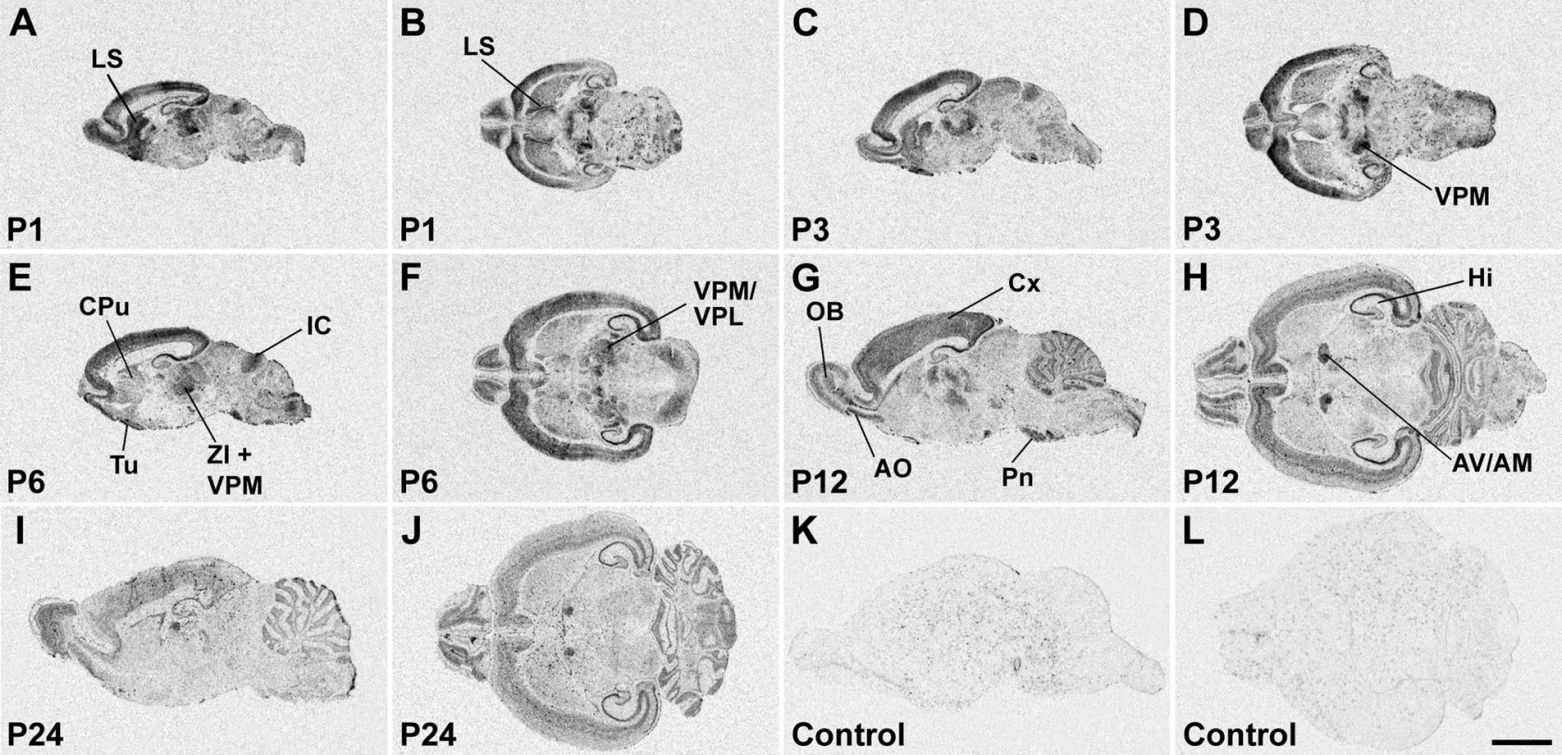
Distribution of SK1 channel subunit mRNA in the CNS at postnatal stages P1, P3, P6, P12, and P24 is shown in X-ray images of sagittal (A,C,E,G,I,K) and horizontal (B,D,F,H,J,L) sections hybridized with an oligonucleotide probe specific for SK1. Strong hybridization signals are present in the anterior olfactory nucleus (AO) and olfactory tubercle (Tu), cerebral cortex (Cx), hippocampal formation (Hi), and several thalamic nuclei. For details on the distribution see Table4. Control sections (K,L) were hybridized in the presence of 100-fold excess cold oligonucleotide, showing a uniformly low background signal. For abbreviations see list. Scale bar = 4 mm.
